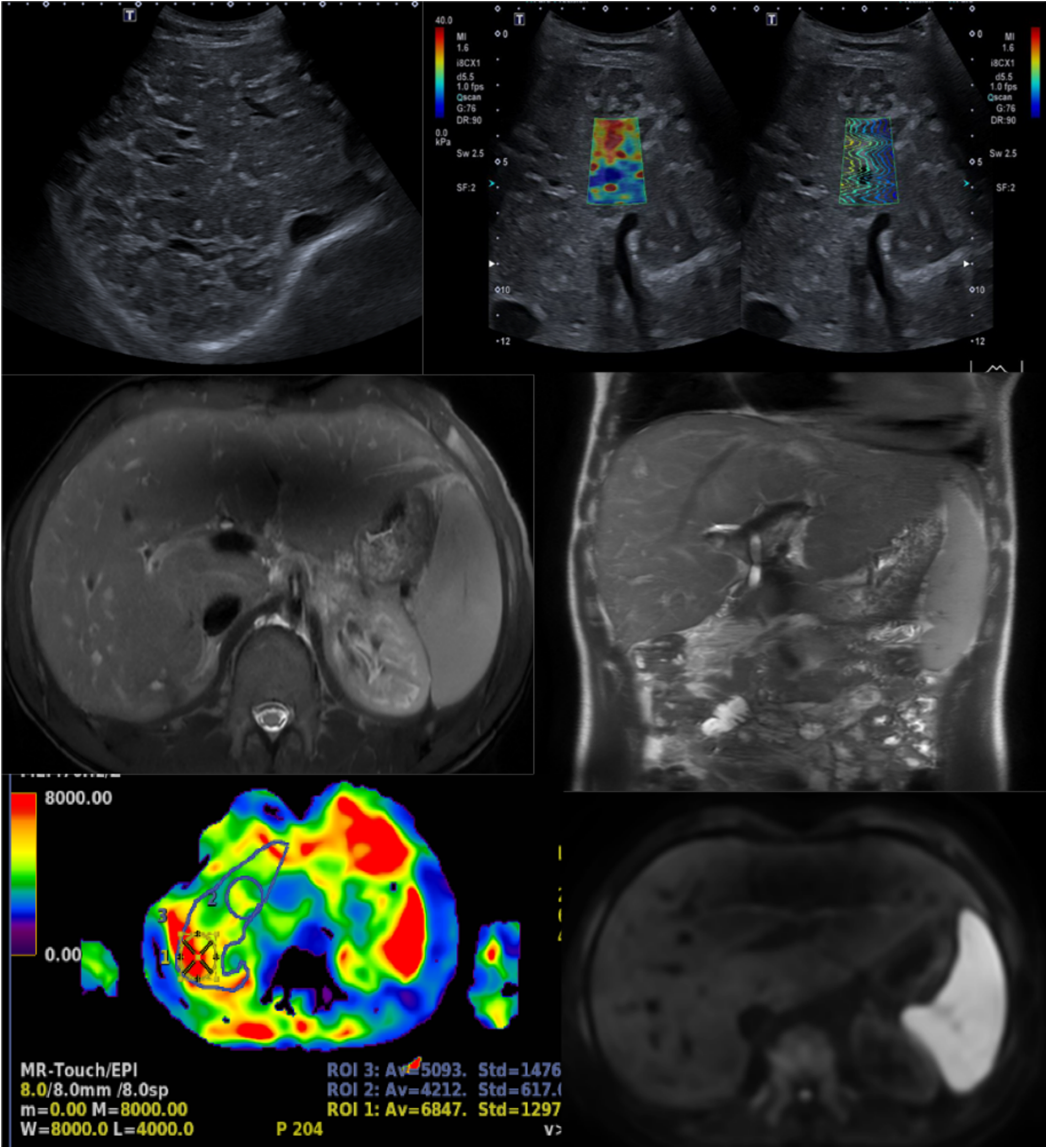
A 17-year-old boy with a hypoplastic left heart complex and Fontan circulation (same patient as in Figure 5). Clinical workup revealed a slightly increased GGT and a newly developed reduced exercise capacity. Ultrasound reveals severe heterogeneous echogenicity (upper left) and increased values of elasticity of 25 kPa (upper right). Abdominal MR T2-weighted coronal image (middle right), with increased size right liver lobe. The liver length is 20 cm and there is a splenomegaly measuring 17 cm in length. Corresponding axial T2-weighted image reveals the reticular peripheral pattern (middle left). Heterogenous diffusion (lower right). MR elastography in an axial plane, measuring increased values of 5.1 KPa (lower left). GGT, gamma-glutamyl transferase.
